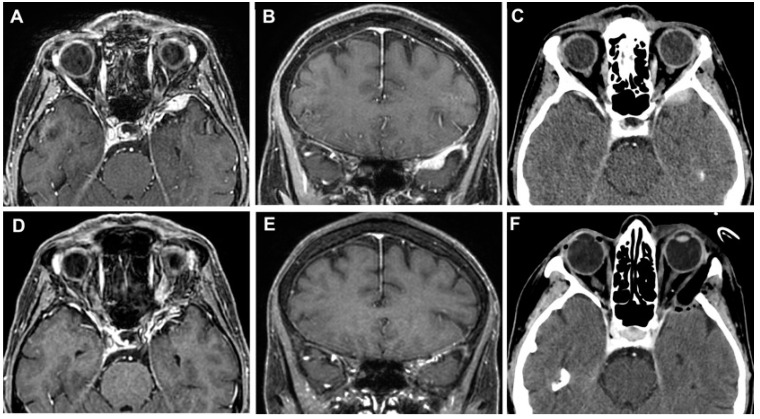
Temporal pole meningioma on preoperative MR images (A,B) and on axial CT scan (C). The post-operative MRI (D,E) and CT scan (F) documented the complete excision of the lesion.
